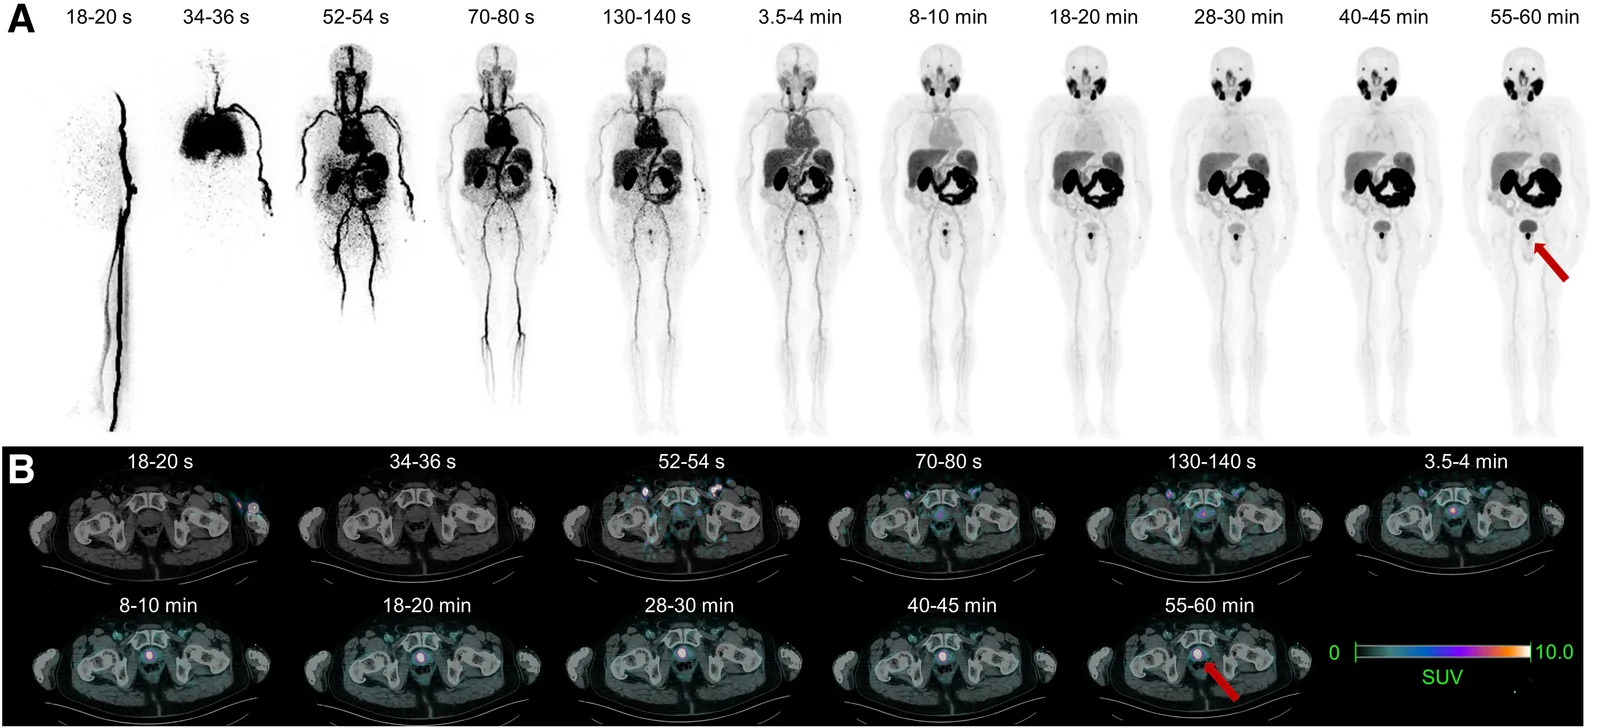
Dynamic total-body PSMA PET. 76-y-old patient with PCa (PI-RADS 2; initial PSA, 19.7; PSA density, 0.82). Representative reconstructed dynamic total-body PET maximum-intensity projection images (A) and transaxial fused PET/CT images (B). Red arrows indicate area of intense uptake in prostate (PRIMARY score 5; ISUP 4 after biopsy; SUV_max_, 55.6).
